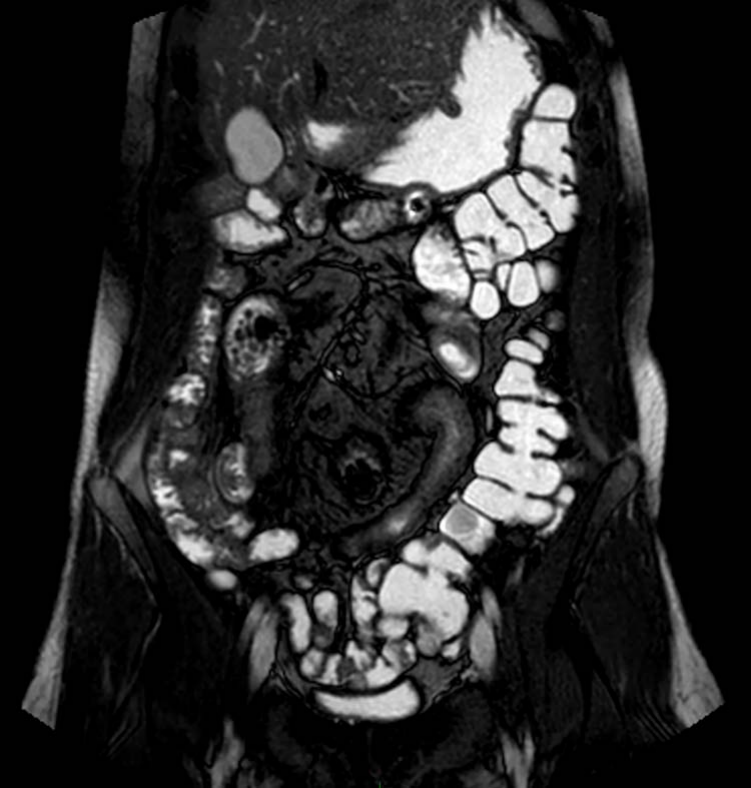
MRI enterography depicting intestinal malrotation and ileal loops in the central abdomen dilated up to 5 cm, consistent with malrotation and a small bowel obstruction.
